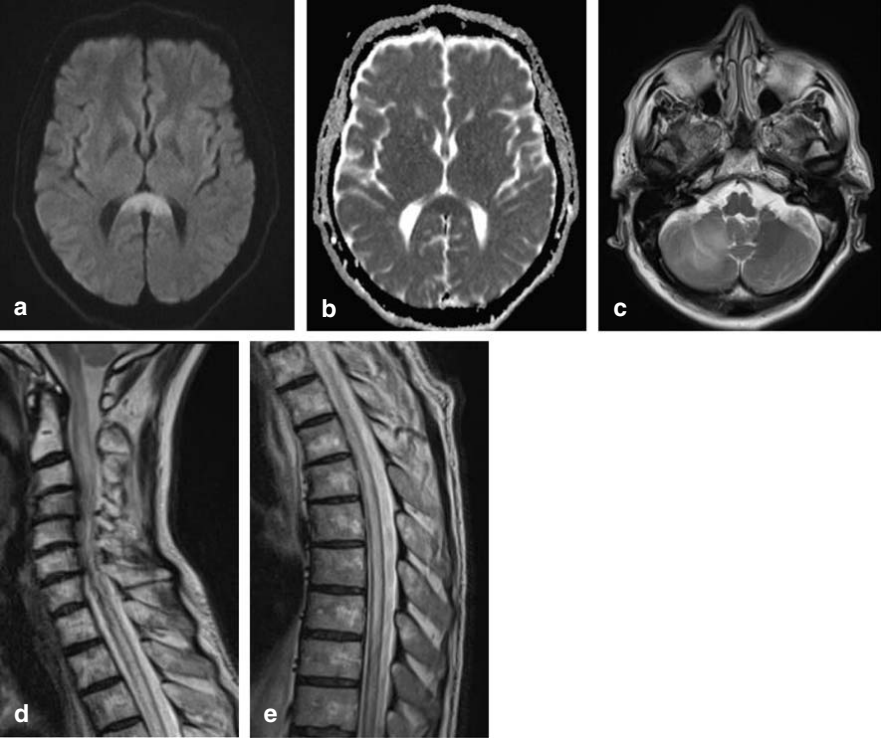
Sixty-four-year-old man with biopsy proven demyelination with some T cell infiltrate and slight secondary axonal injury, consistent with ADEM. Axial DWI (a) and ADC (b) show large splenial lesion with diffusion restriction, without enhancement (not shown). Axial T2 (c) shows lesion in the right cerebellar hemisphere, with central punctiform enhancement (not shown). Swelling of the entire spinal cord shown on sagittal T2 (f, g)
